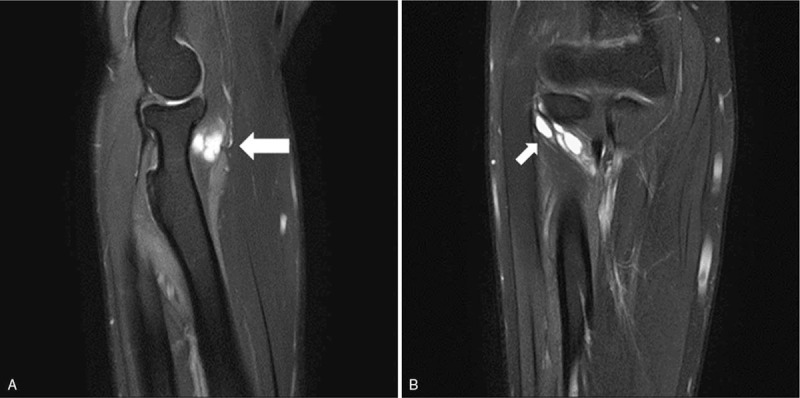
Preoperative magnetic resonance imaging (MRI) findings. A multilobulated cystic lesion anterior to the radial head (arrows) was shown in the sagittal (A) and coronal (B) views of MRI.
